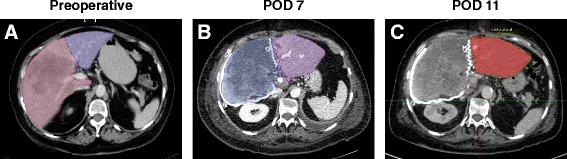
Pre- and postoperative CT-scan of the liver. a Preoperative CT-scan depicting the tumor lesion within the right liver lobe (marked pink) and the resulting future liver remnant (FLR; marked purple). b CT-volumetry 7 days after the first step of ALPPS already resulted in a significant increase of the FLR. The extended right liver lobe (wrapped in a silicone matting) meanwhile showed signs of necrosis following ligation of the right portal vein. c CT-volumetry 11 days after the first step of ALPPS showed further growth of the FLR (marked red). POD = postoperative day
